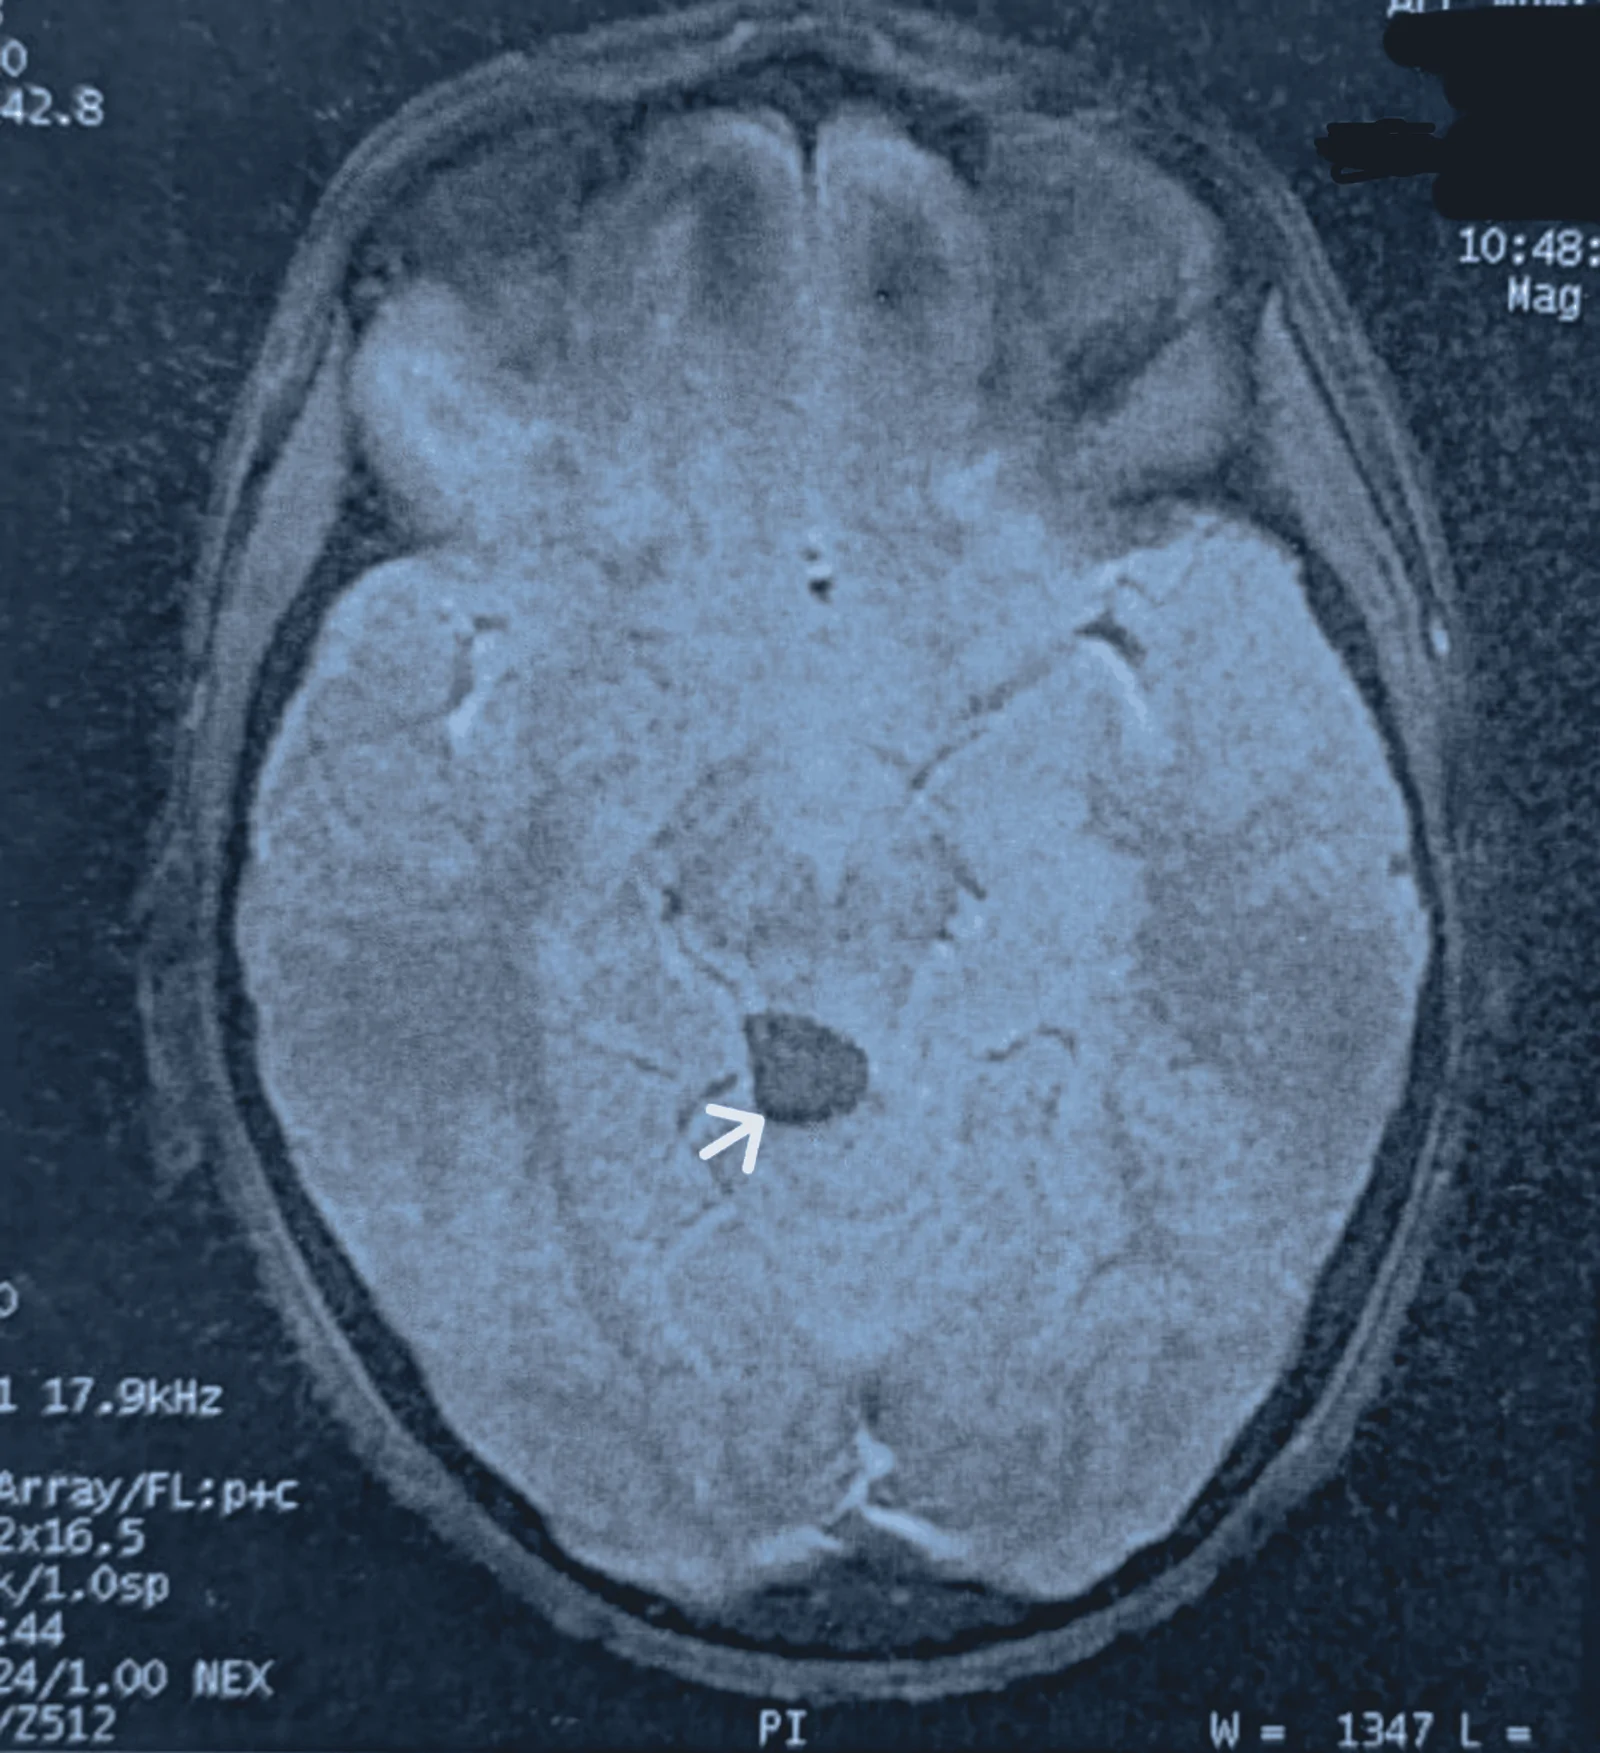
Axial T1 FAT SAT (fat-saturated) images magnetic resonance imaging brain image showing quadrigeminal cistern lipoma on the right side (white arrow)
